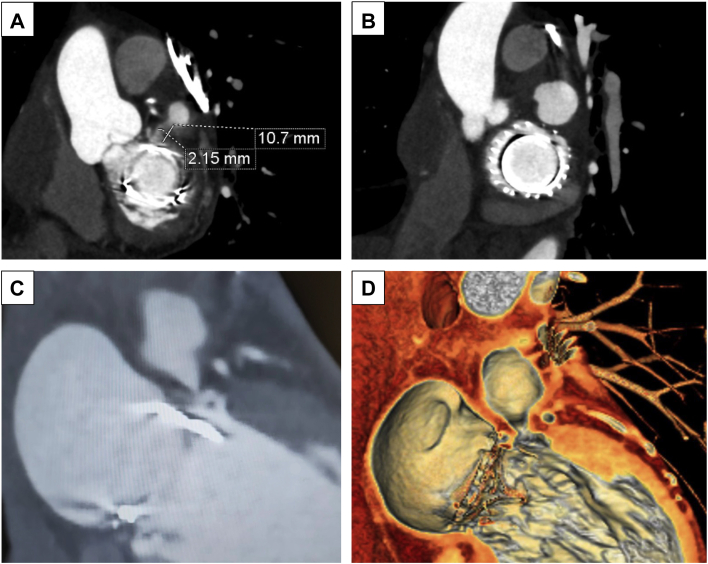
Computed tomography images show (A) a narrow communication between the left ventricle and an aneurysm sac and (B, C) an aneurysm sac adjacent to the mitral prosthesis. (D) Three-dimensional reconstruction of the aneurysm.
